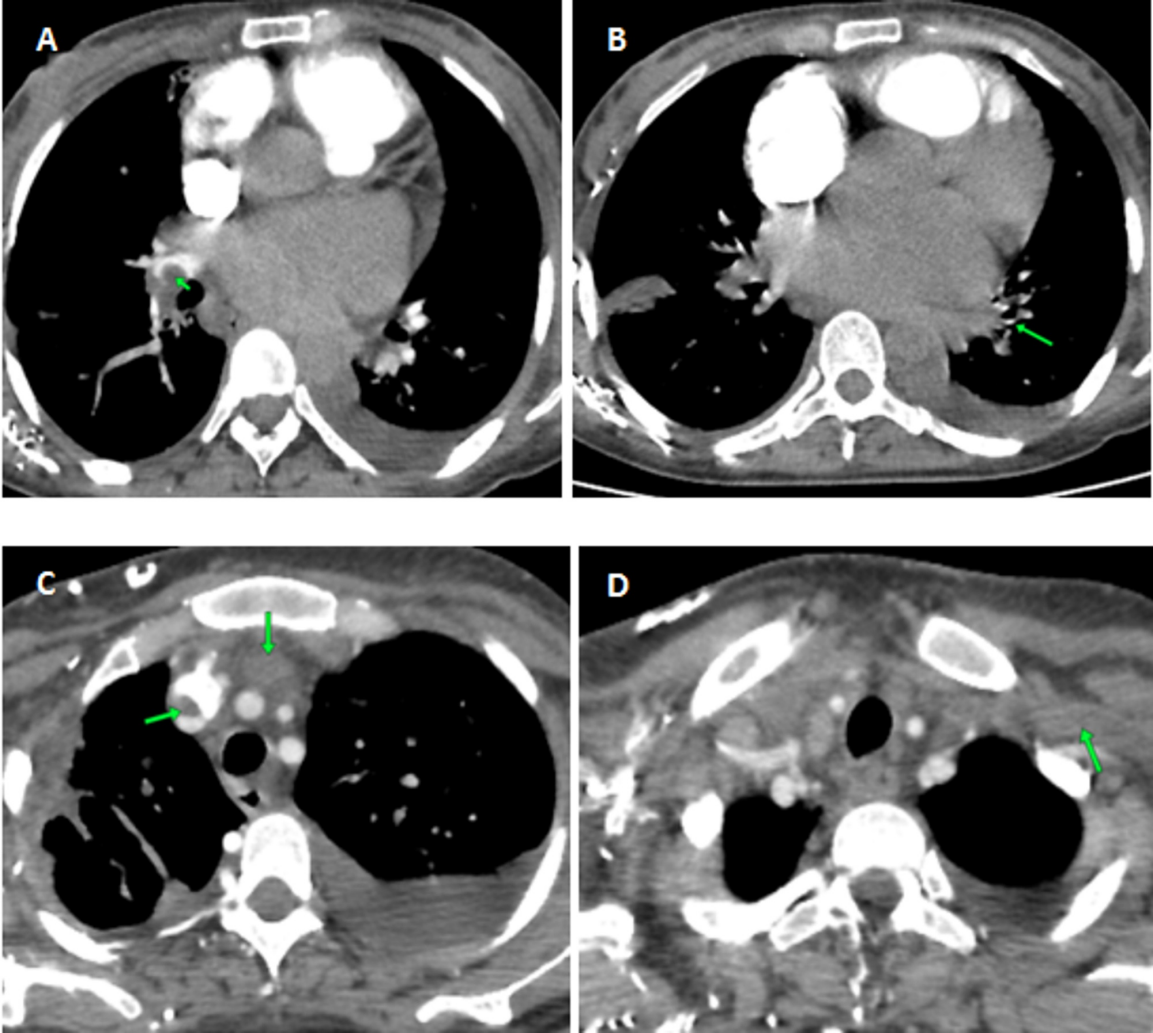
Chest CT angiogram during the second hospitalization A: right pulmonary embolism (arrow). B: pulmonary embolism of left segmental branches (arrow). C: deep vein thrombosis of the innominate venous trunk and the origin of the superior vena cava (arrows). D: deep vein thrombosis of the subclavian vein (arrow) CT: computed tomography
